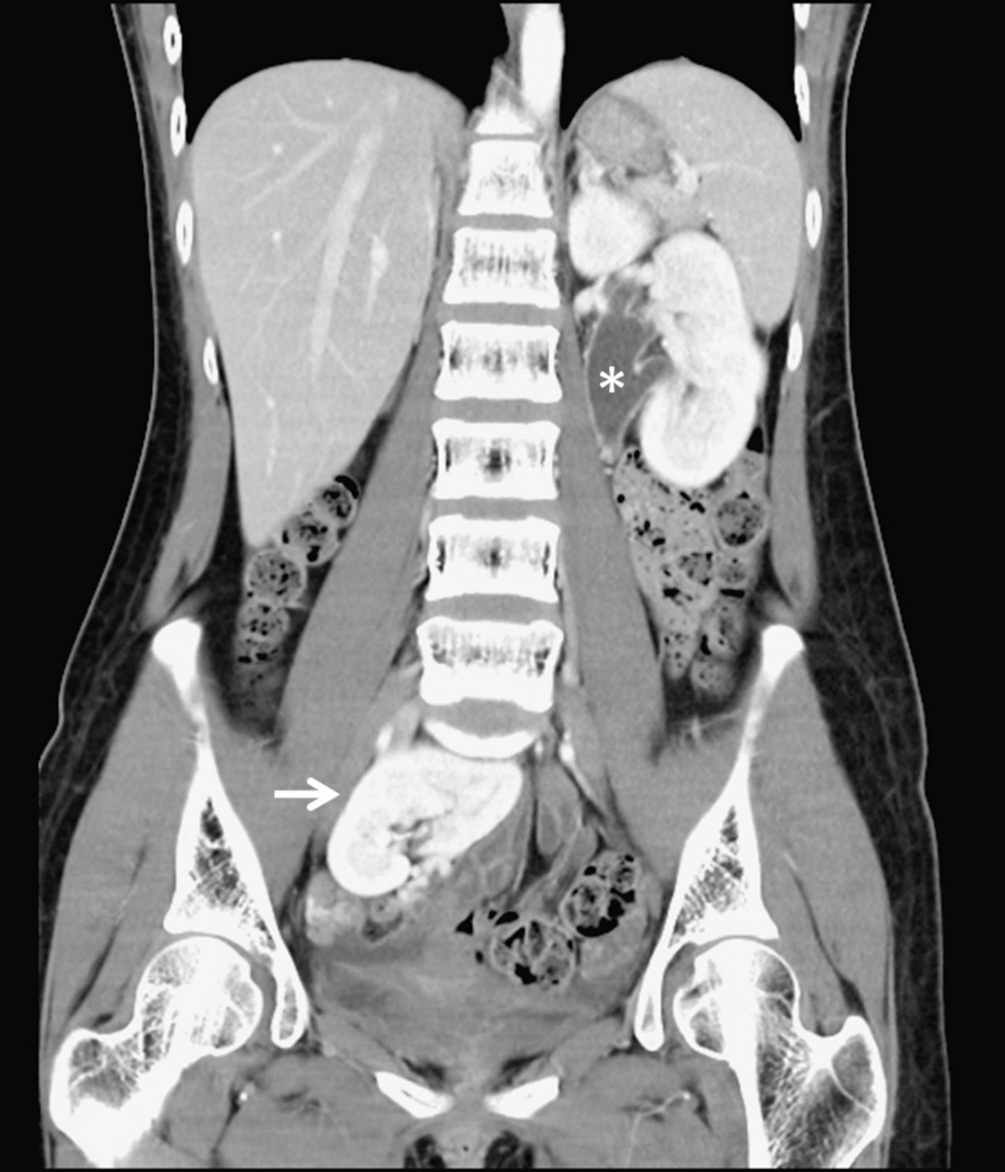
Abdomen-to-pelvis computed tomography with contrast showing the right ectopic pelvic kidney (arrow) and left dilated renal pelvis (✽)
